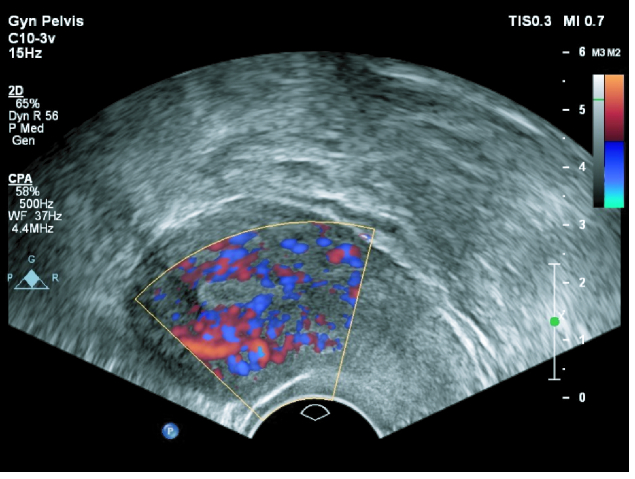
Endometrial perfusion by power Doppler ultrasound.
